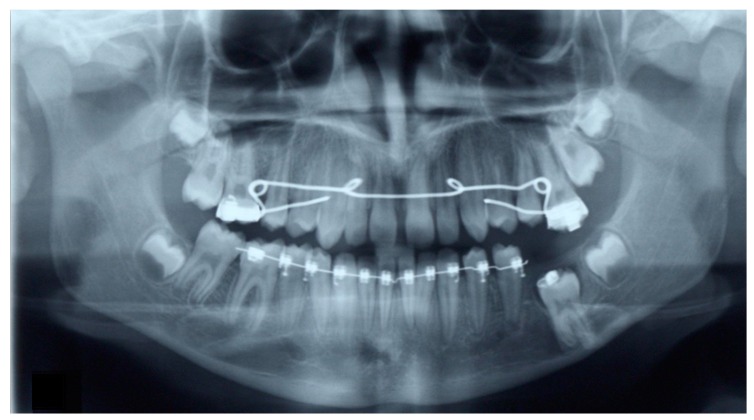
Panoramic radiograph two months after the surgical exposure of the first permanent mandibular molar. Note the reduction in size of the former cystic cavity and the more favorable axis of the impacted tooth.
